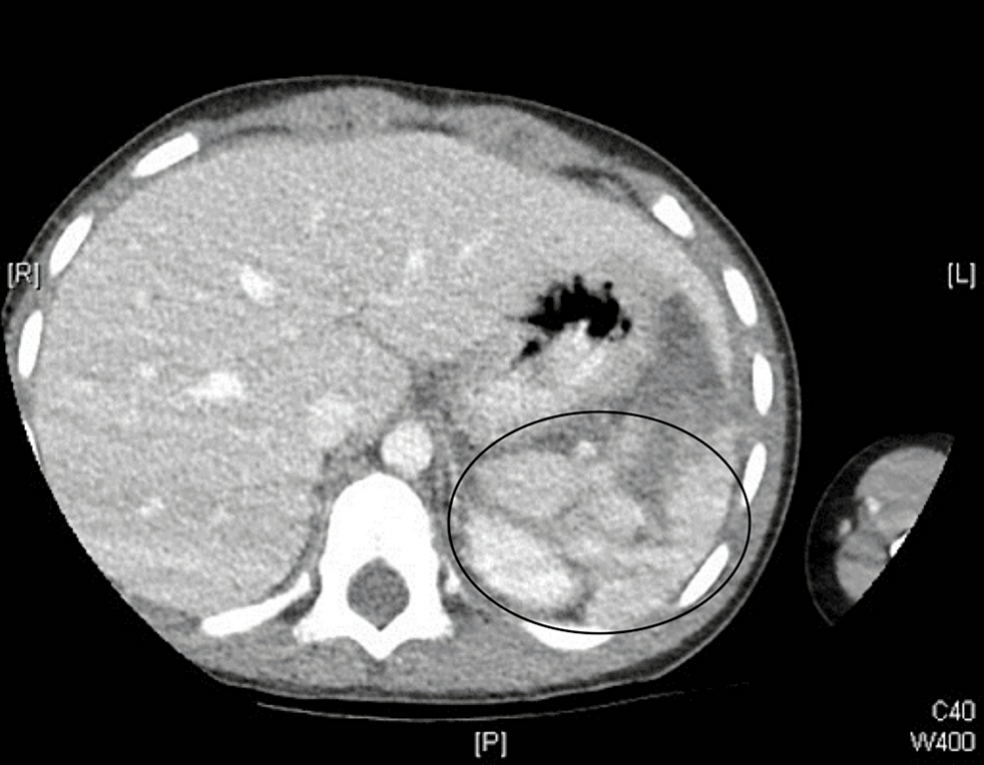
CT abdomen (axial) consistent with splenic laceration
